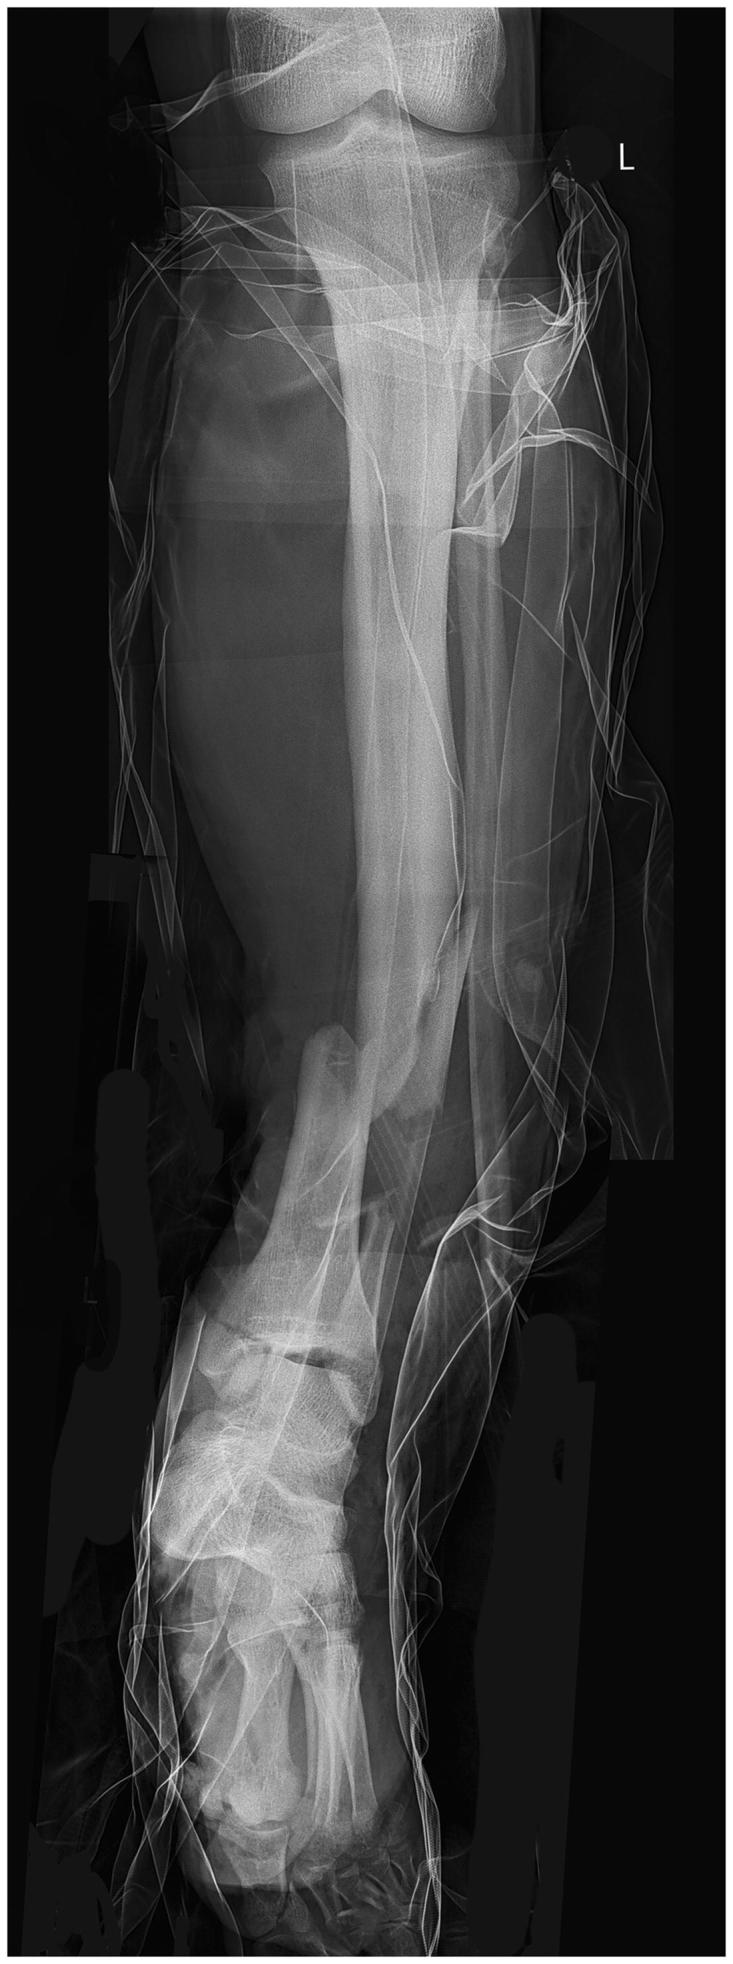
X-ray photo of the bone fractures.
